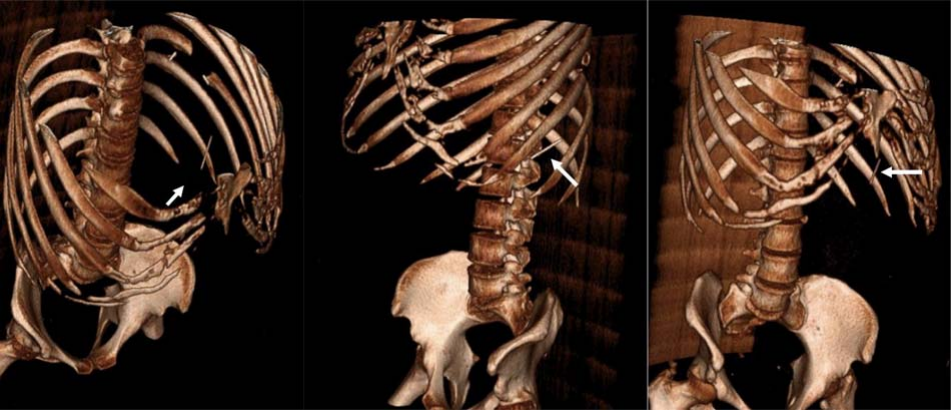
Three-dimensional reconstruction CT scan displaying the foreign body (white arrow) in three different orientations.
